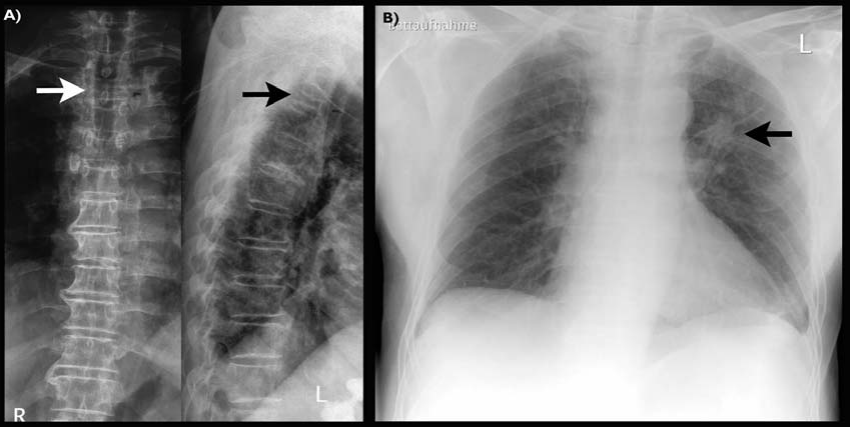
Conventional radiographs of the thoracic spine and the chest on initial presentation. (A) Compression fracture of the third thoracic vertebra (arrows). (B) Solitary pulmonary nodule of 2.5 cm in diameter of the left upper lobe (arrow), in posterior-anterior and lateral projection, respectively.
